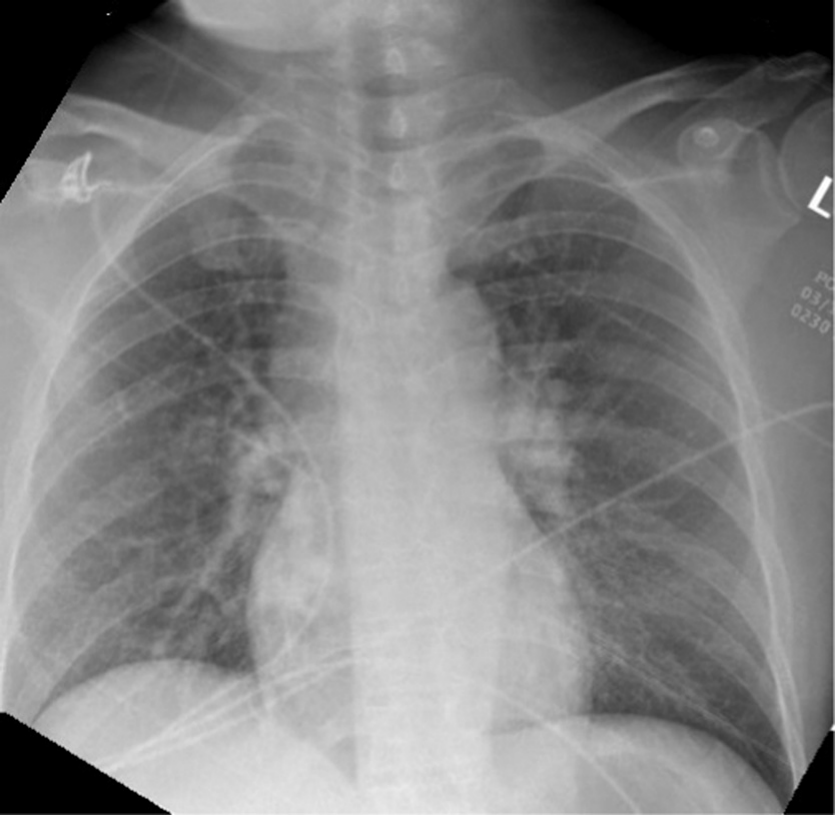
One view anterior-posterior chest radiograph obtained due to patient’s complaint of chest tightness during ascent to high altitude and tachypnea on presentation. Pulmonary vascular congestion is seen throughout. There is no evidence of pneumothorax, cardiomegaly, or consolidation.
